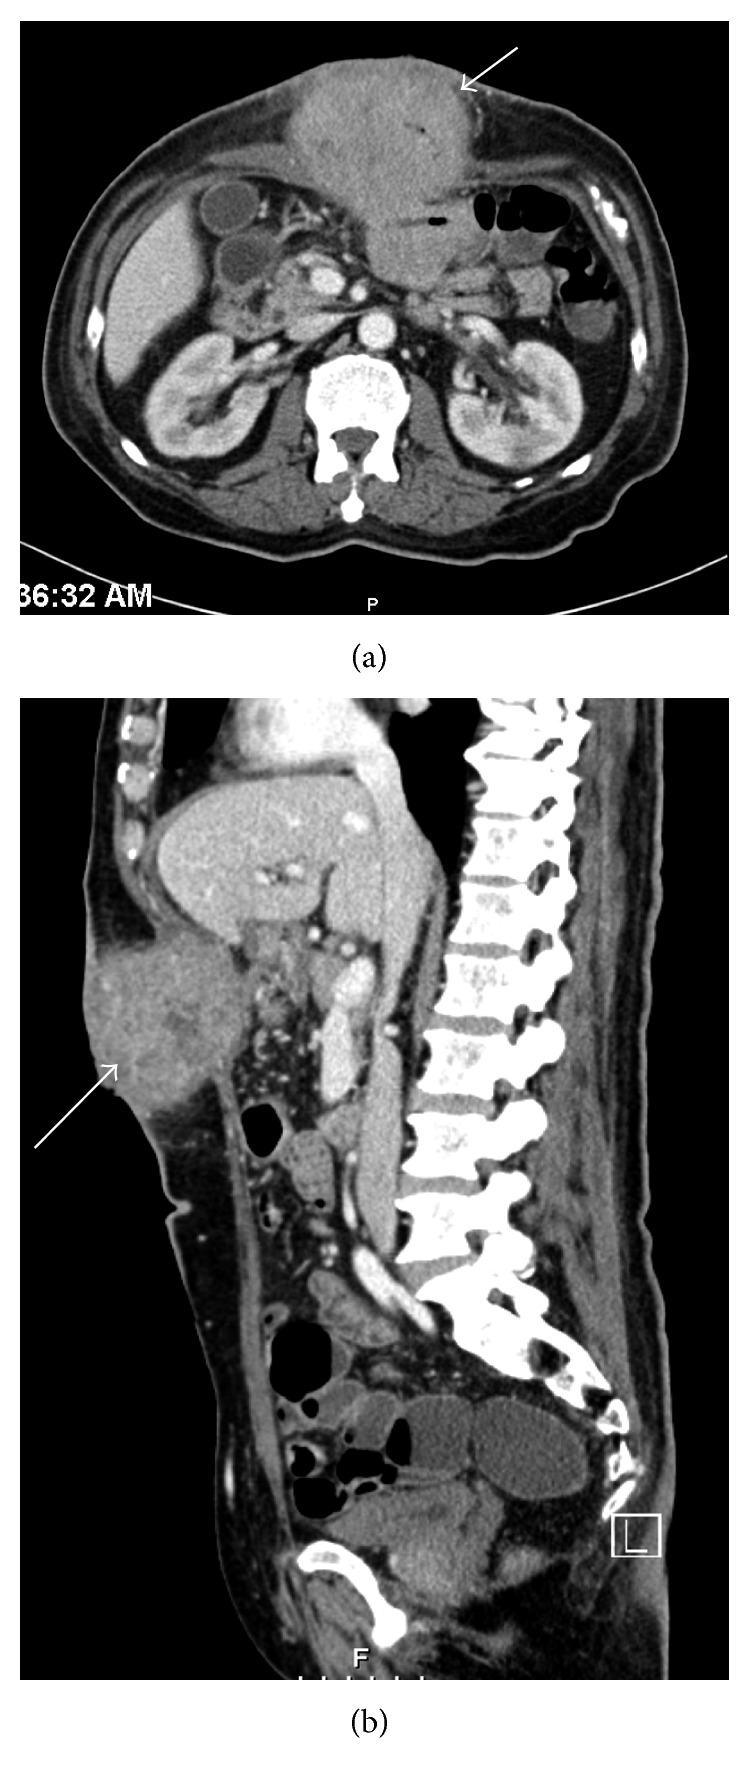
CT scan showing the mass, extending to the adjacent muscles and penetrating the abdominal wall.
